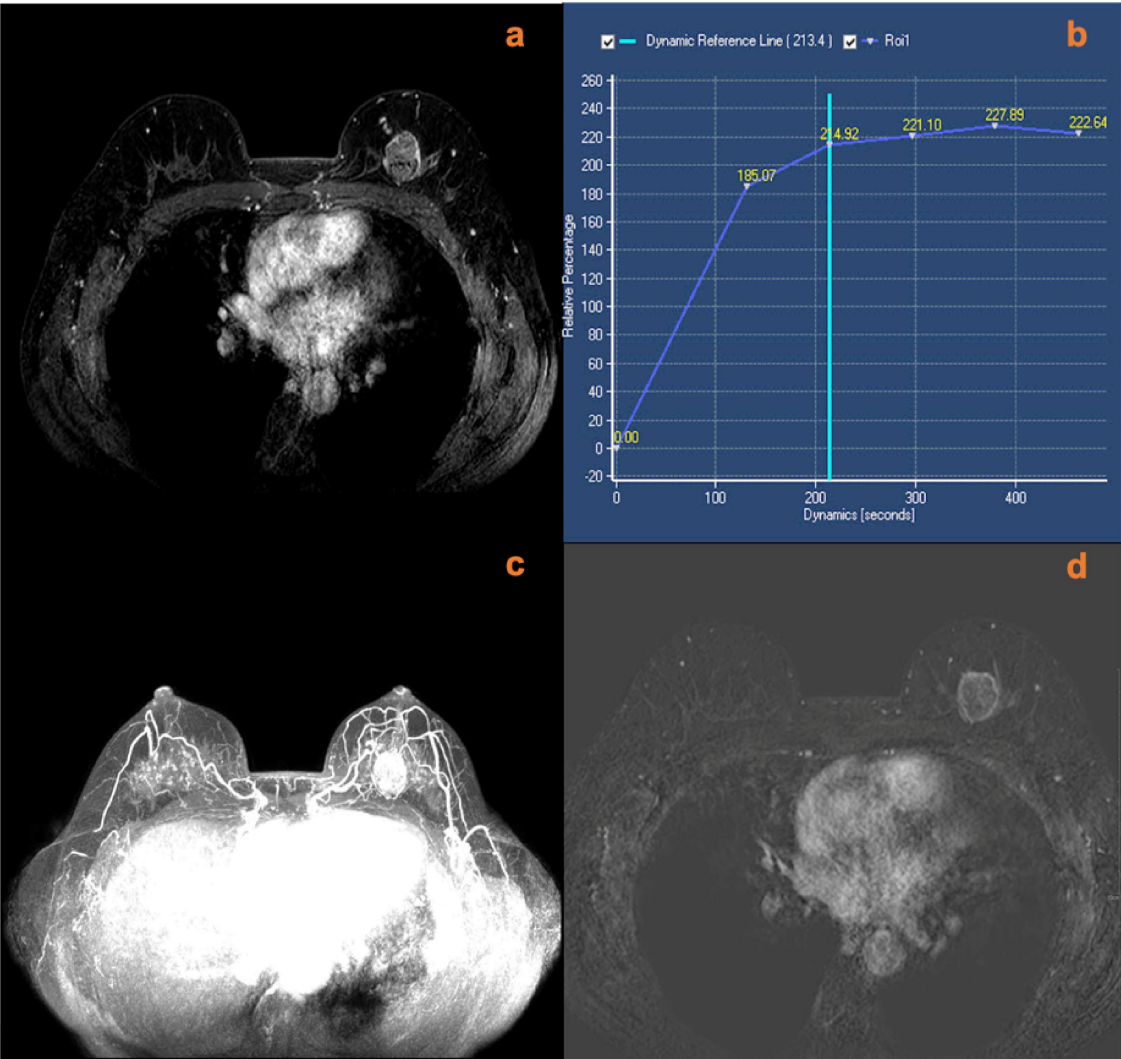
A 49-year-old female was identified with a left breast mass lesion for 3 months. MRI (A, contrast-enhanced axial; B, time signal intensity curve; C, maximum intensity projections image; D, subtraction image) shows a left breast mass lesion with an irregular margin and plateau enhancement. The lesion also increased ipsilateral breast vascularity and had a positive AVS sign. The KS of the lesions was 5, while KS^*^ was 8. Postoperative pathology confirmed that the lesion was an invasive ductal carcinoma.
